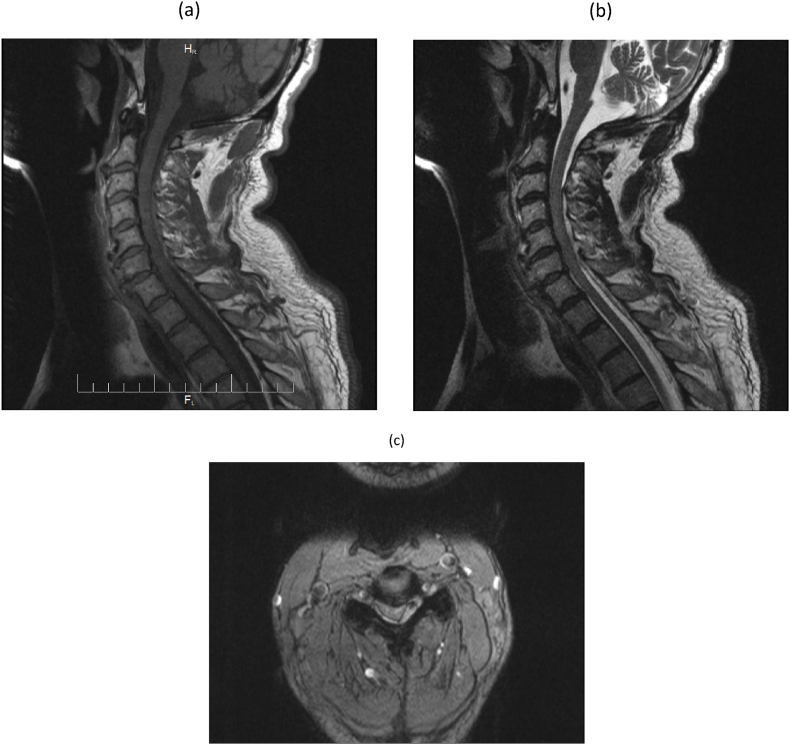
(a) Sagittal T1-weighted image of the cervical spine shows a fluid collection (arrow) in the posterior spinal canal with slight hyperintensity compared with the spinal cord. There is mass effect on the cervical dura mater and spinal cord with anterior displacement. (b) Sagittal T2-weighted image of the cervical spine shows a fluid collection (arrow) in the posterior spinal canal with isointense fluid collection along the posterior spinal canal, with displacement of the dura by the posterior epidural collection. (c) Axial T2-weighted image at the level of C4C5 shows the posterior fluid collection (white arrow), which appears slightly isointense to the spinal cord with circumferential hypointense hemosiderin deposits (black arrow), and results in external mass effect on the dura mater and displacement of the spinal cord.
